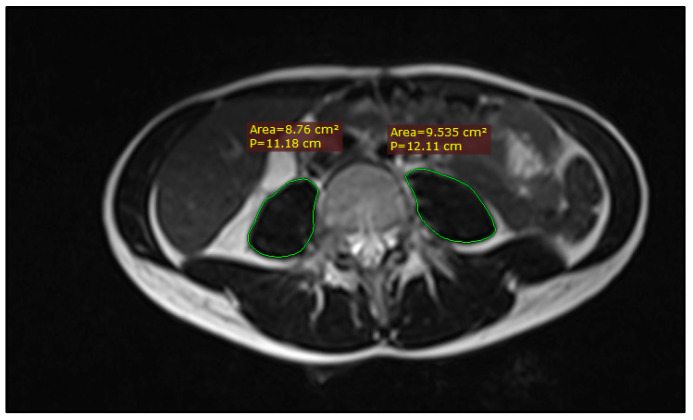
Example of PMA measuring on an axial slice of a T2-weighted MRI image at the level of L4.
